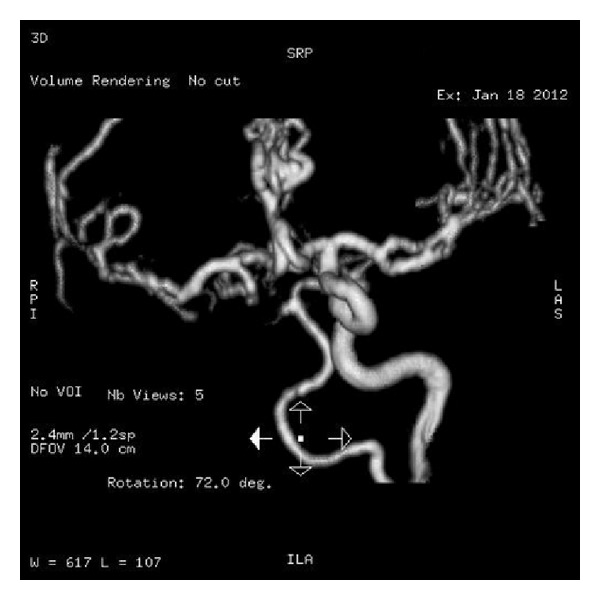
Brain Angio MRI showing occlusion of left internal carotid.
